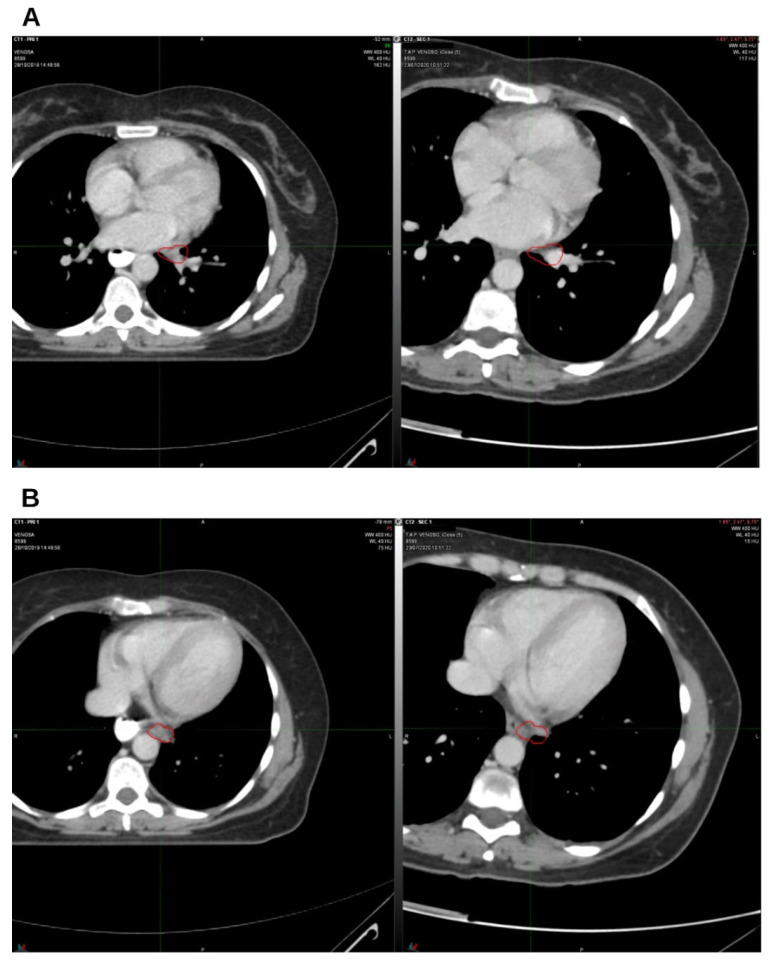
The third follow-up at 9 months off. On the left, CT from October 2019, pre-radiation therapy treatment; on the right, CT from July 2020. (A) Hilar lymph node; (B) left para-aortic lymph node. The indicated region highlights the boundary of the treated target.
